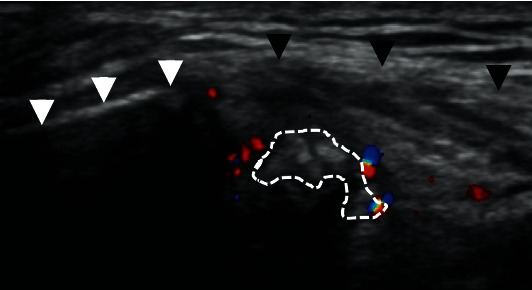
Color Doppler sonogram showing fragmented calcification (dotted line) with hypervascularity, the anterior acromion (white arrowheads), and the deltoid (black arrowheads).
